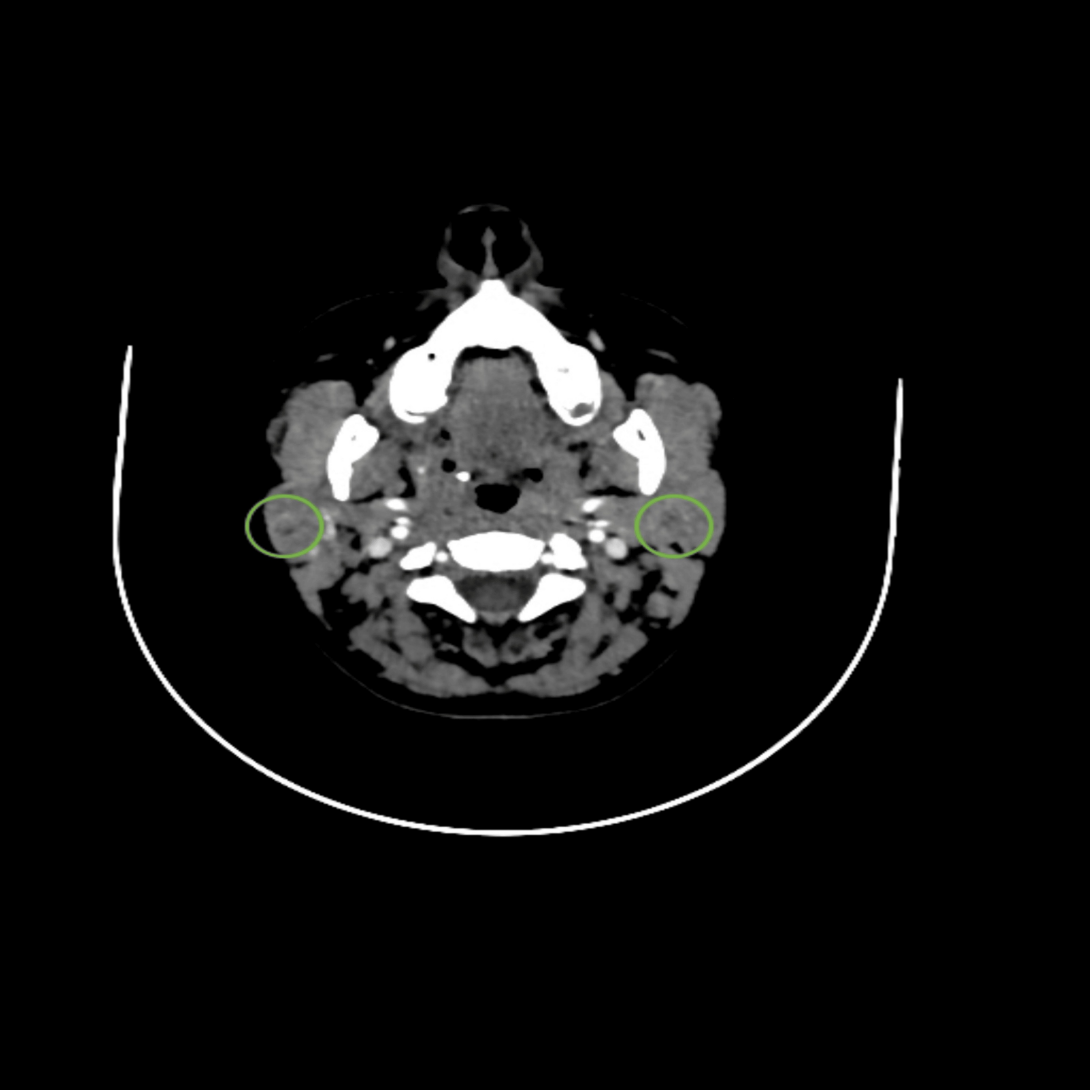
Postcontrast CT showing cystic changes in parotid glands bilaterally. The cystic changes are highlighted by the green circles.
